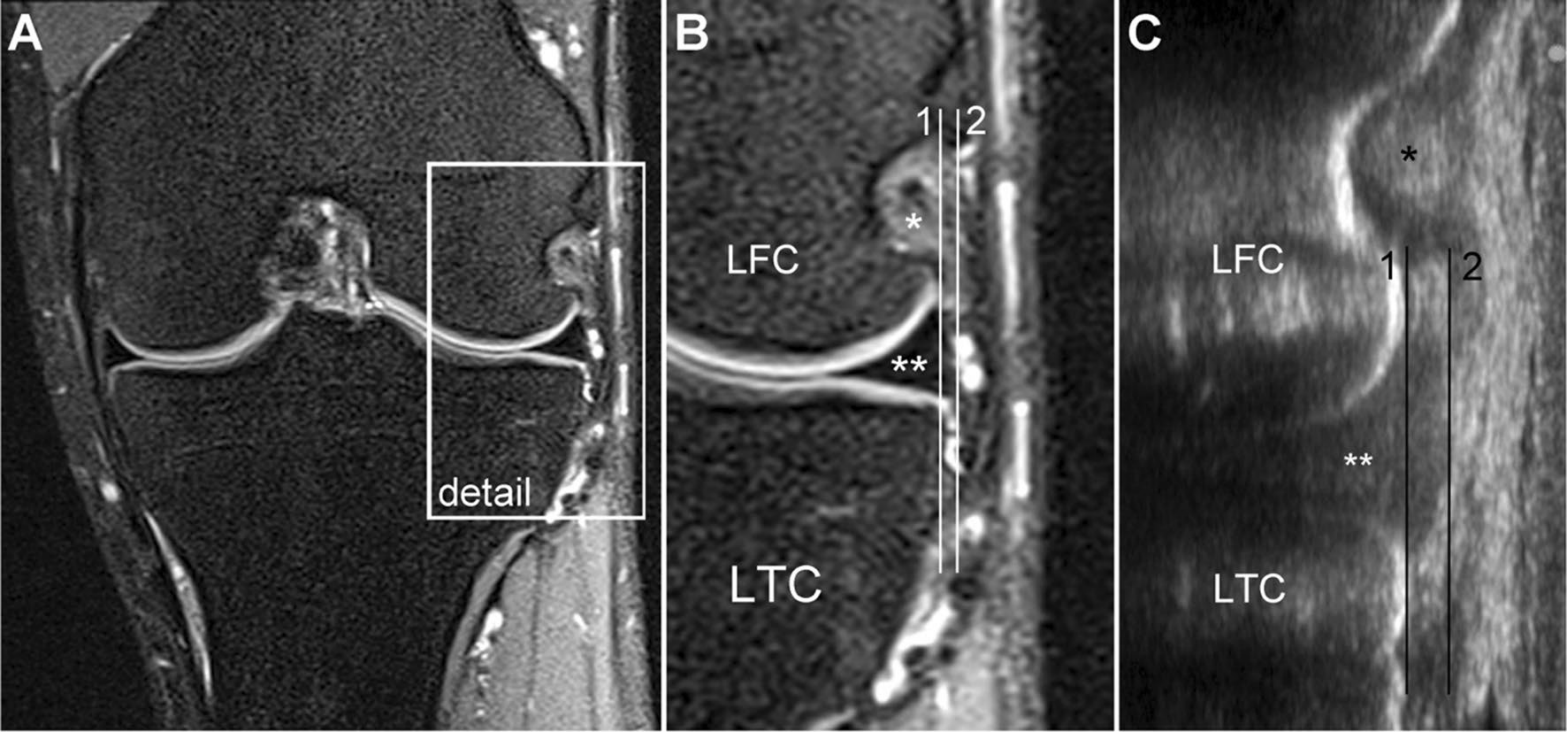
Measurement technique. a MRI overview. Detail of the MR image (b) and ultrasound slice (c) for meniscal extrusion measurement. Lateral meniscal extrusion is defined as the horizontal distance between line 1 and line 2. Line 1, running along the lateral tibial cortex margin. Line 2, running through the most peripheral lateral meniscus margin and parallel to line 1. *LFC* lateral femoral condyle. *LTC* lateral tibial condyle. *, popliteus tendon. , lateral meniscus
